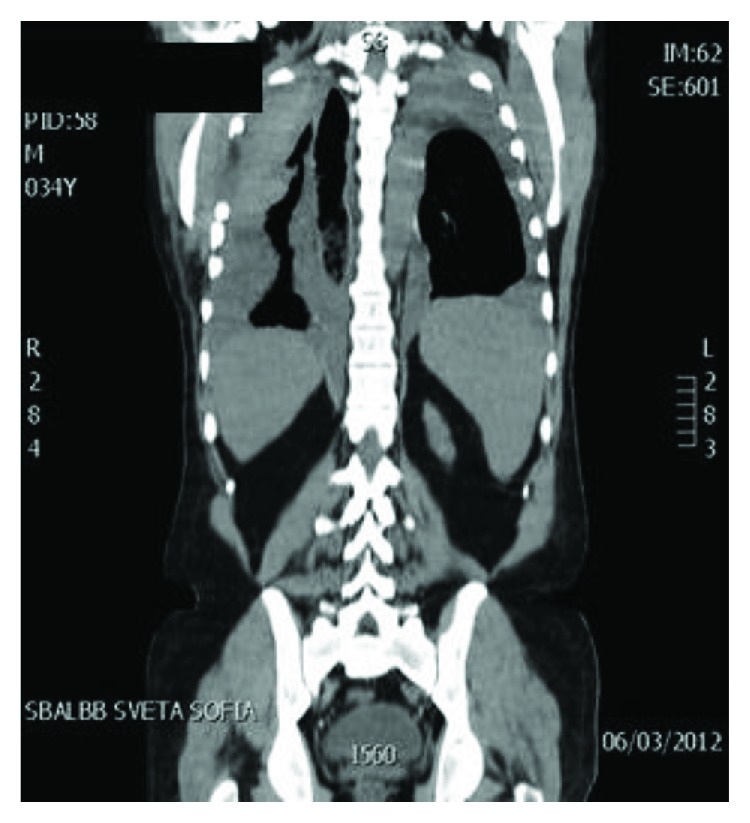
Chest CT—a massive pleural effusion on the right side and a small one on the left.
